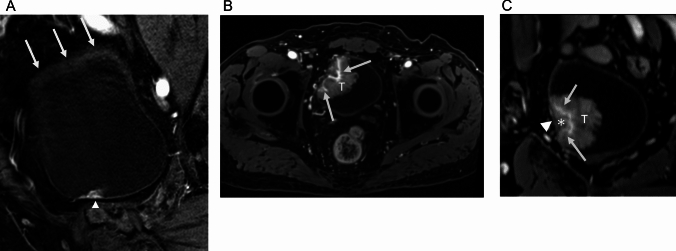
Cases of non-muscle invasive bladder cancer. A Dynamic Contrast-Enhanced MRI (DCE-MRI) acquisition using Cartesian method at 60 s post-contrast injection. The DCE-MRI displays significant intestinal motion artifacts on the bladder’s superior wall (arrows). The tumor located on the inferior wall of the bladder allows for clear evaluation. Preservation of inner layer enhancement at the tumor base (arrowhead) supports the diagnosis of non-muscle invasive bladder cancer. Pathological findings from transurethral resection of the bladder tumor (TURBT) confirmed a T1 high-grade cancer. B DCE-MRI acquisition using GRASP method, axial section at 60 s post-contrast injection. The tumor is observed on the right wall of the bladder (labeled as T), with retention of inner layer enhancement indicated (arrows). C Reconstructed cross-sectional image where tumor (labeled as T) intersects with muscle layer (arrowhead). This reconstruction facilitates a detailed assessment of the inner layer enhancement (arrows) and the stalk (marked by *). Pathological examination from TURBT identified the tumor as Ta low grade
